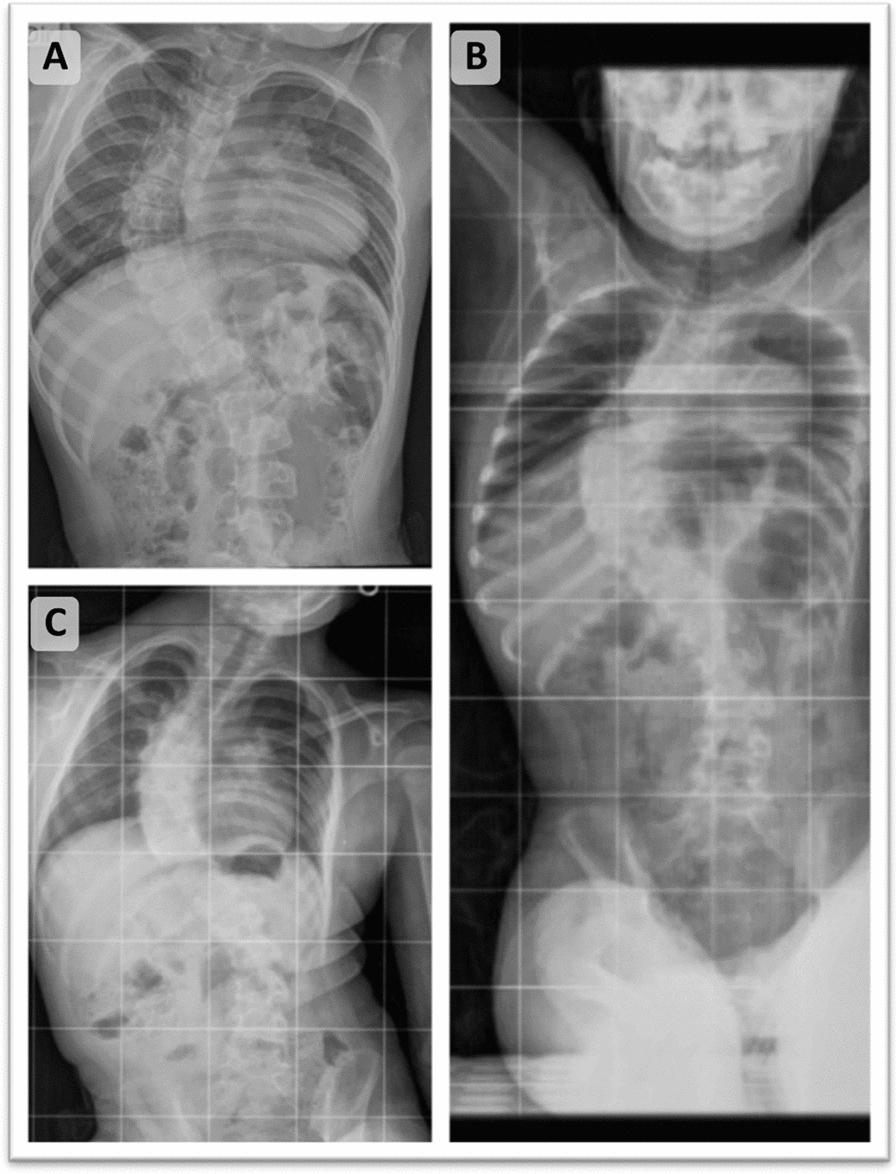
A–C* Spinal X-ray: pronounced dorsolumbar scoliosis with dorsal convexity to the right and lumbar to the left, with the dorsal curvature being the primary one associated with associated with female-restricted X-linked intellectual development disorder 99. *All panels are from the same examination
